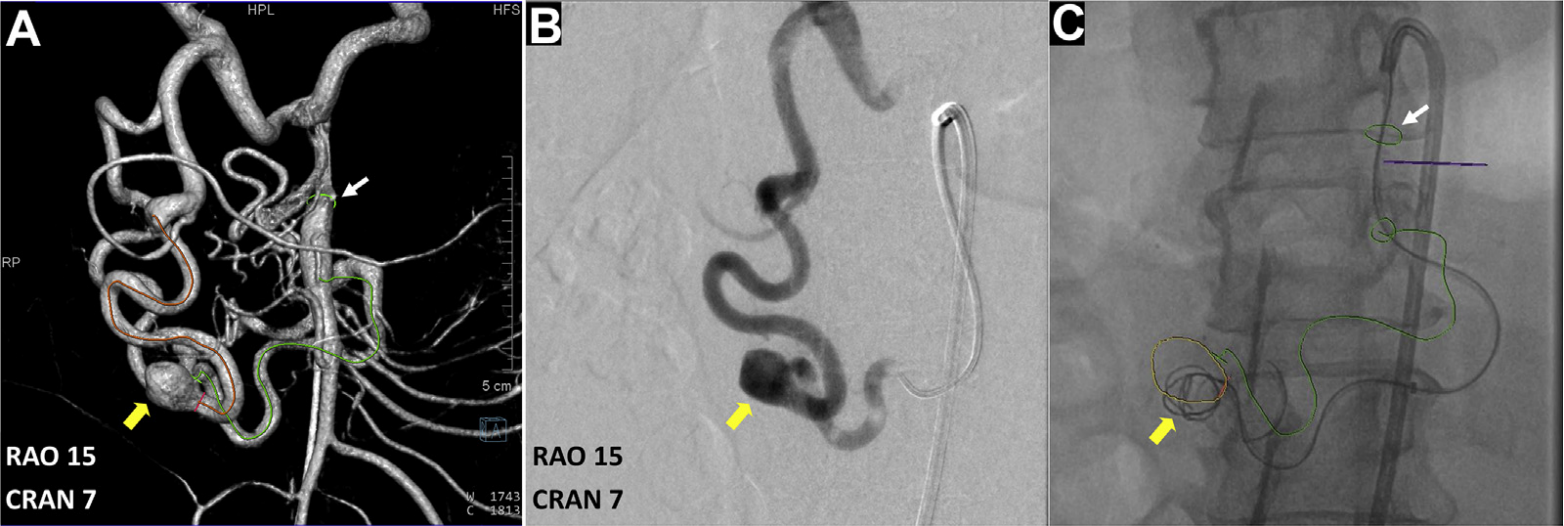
A, Three-dimensional (3D) volume rendered reconstruction of cone-beam computed tomography angiography (CBCTA) with ostia and centerlines of inflow and outflow parent vessels of the aneurysm marked electronically (*green circle* and *green centerline* and *orange circle* and *orange centerline*, respectively). The optimal C-arm working projection of right anterior oblique (*RAO*) 15°/cranial (*CRAN*) 7° angles was selected based on the CBCTA images and 3D annotated vascular landmarks. B, Two-dimensional (2D) angiographic image of the aneurysm acquired at the optimized working projection, demonstrating inflow from the superior mesenteric artery (SMA) and outflow from the aneurysm sac. C, Snapshot of CBCTA-fluoroscopy image fusion guidance during coil embolization showing overlay of vascular landmarks such as the origin of the SMA (*white arrow*), origin/centerline of inflow vessel (*green*), leading into the aneurysm (marked in *yellow outline* and indicated by *yellow arrow*).
